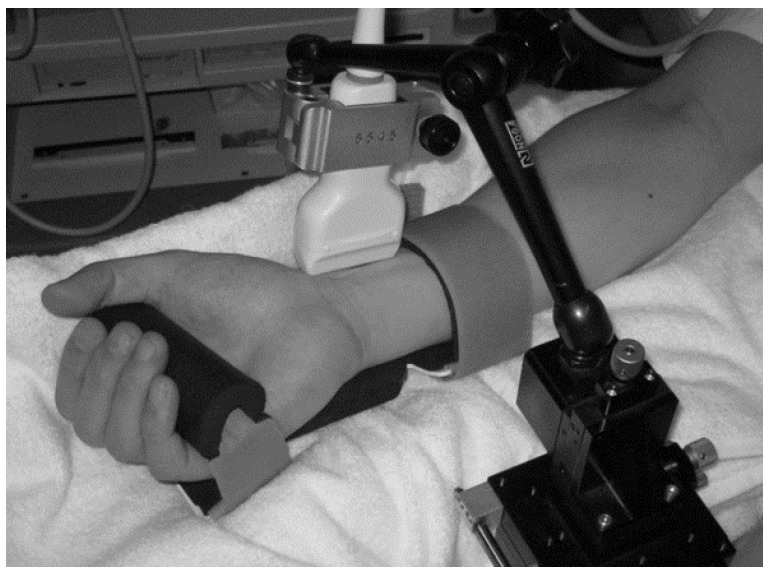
Ultrasonography measurement of radial artery using a special probe holder.
